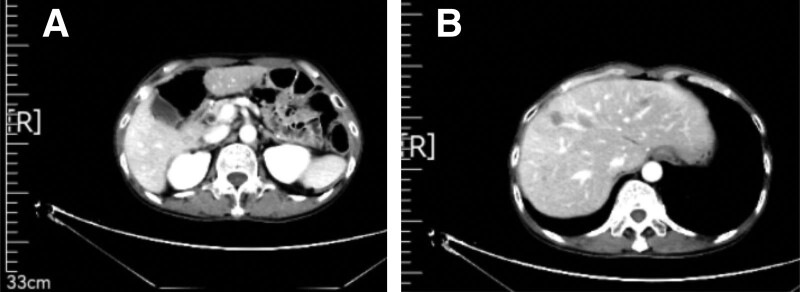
April 2, 2022, CT showed that compared to the previous CT scan of the abdomen and pelvis on December 30, 2021, there were new liver S6 and S7 segment nodules, which were considered metastatic. CT = computer tomography.
